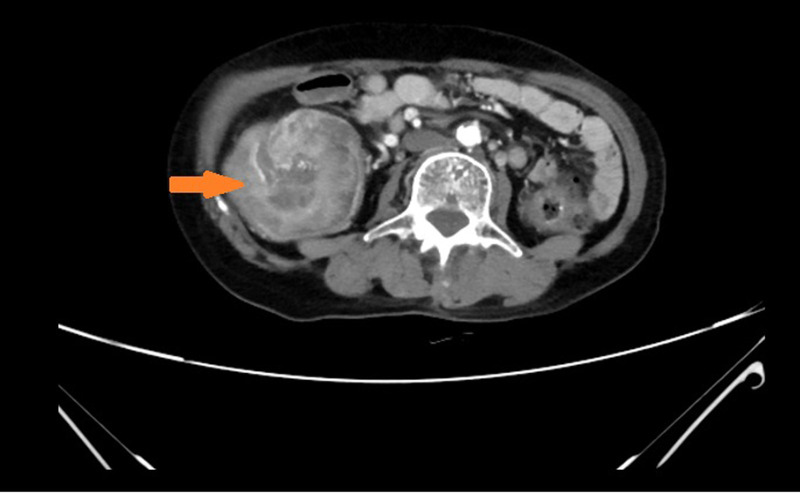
Computed tomography revealed ascending colonic intussusception.
